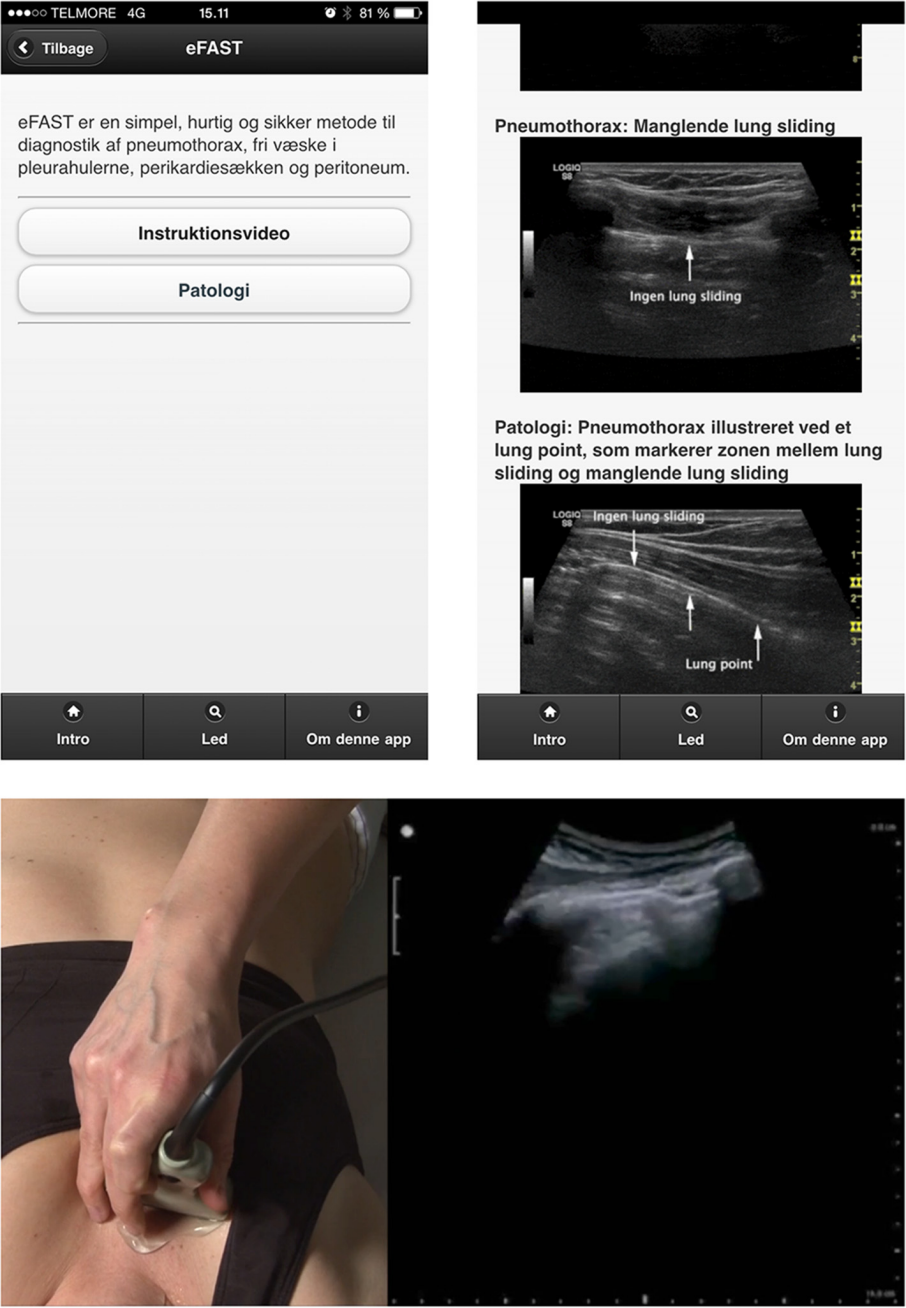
Acute Ultrasound App: Example of app overview of eFAST (top, left) and examples of how to detect pathology (top, right). Example of an instructional video (bottom)
